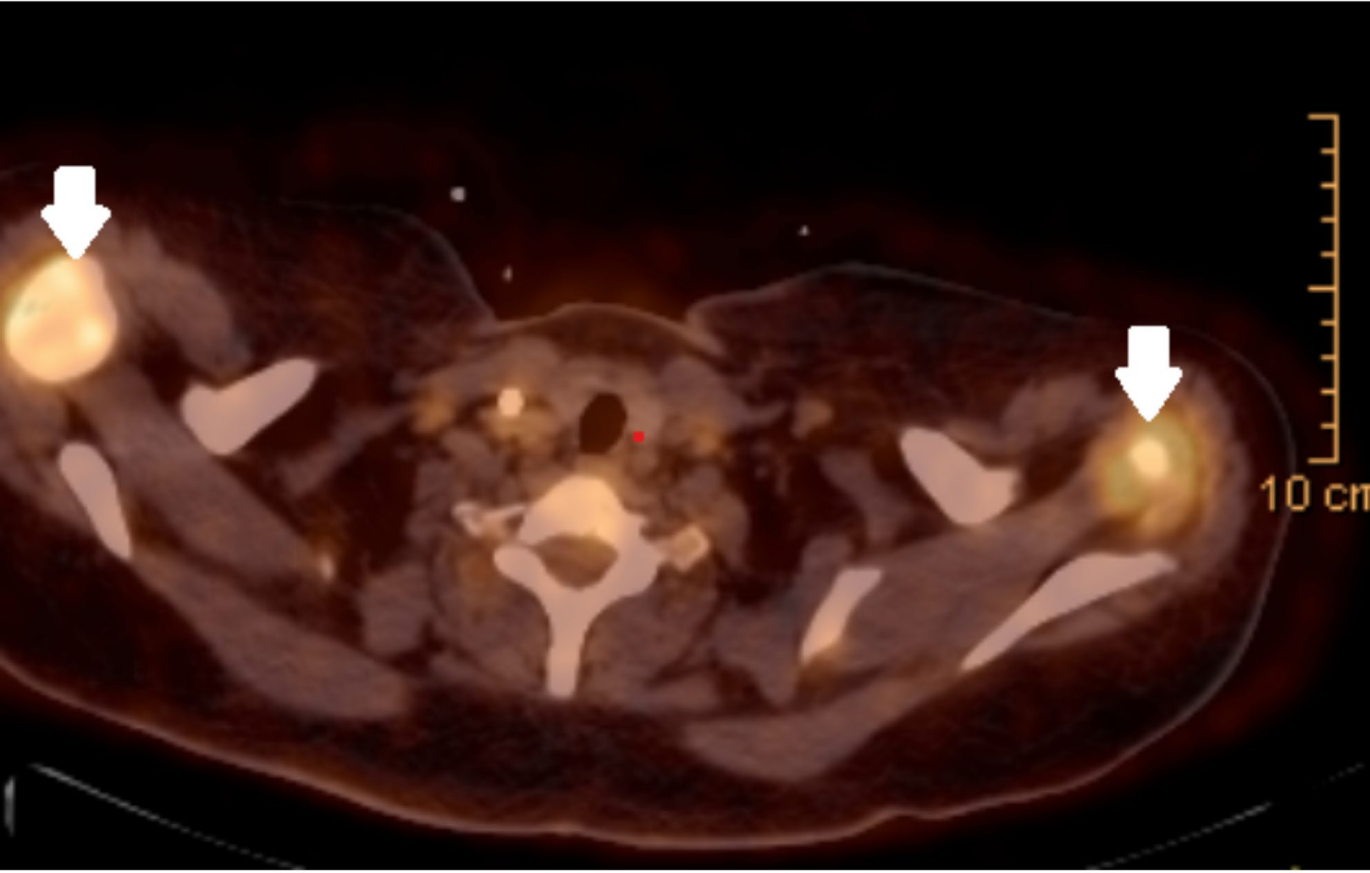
PET-CT showing enlarged axillary lymph nodes with increased FDE uptake
